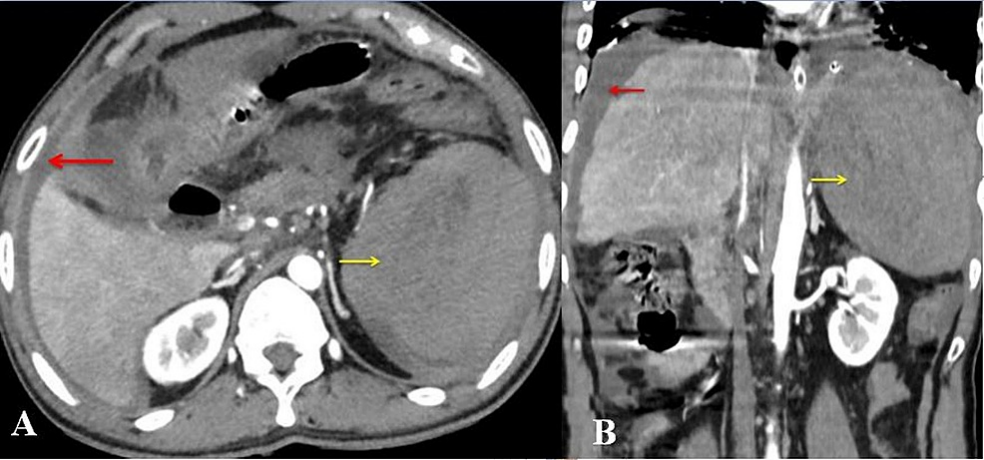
Computed tomography showing arterial phase showing peri-splenic hematoma (yellow arrow) and free fluid abdomen (red arrow). (A) Axial view. (B) Coronal view.
